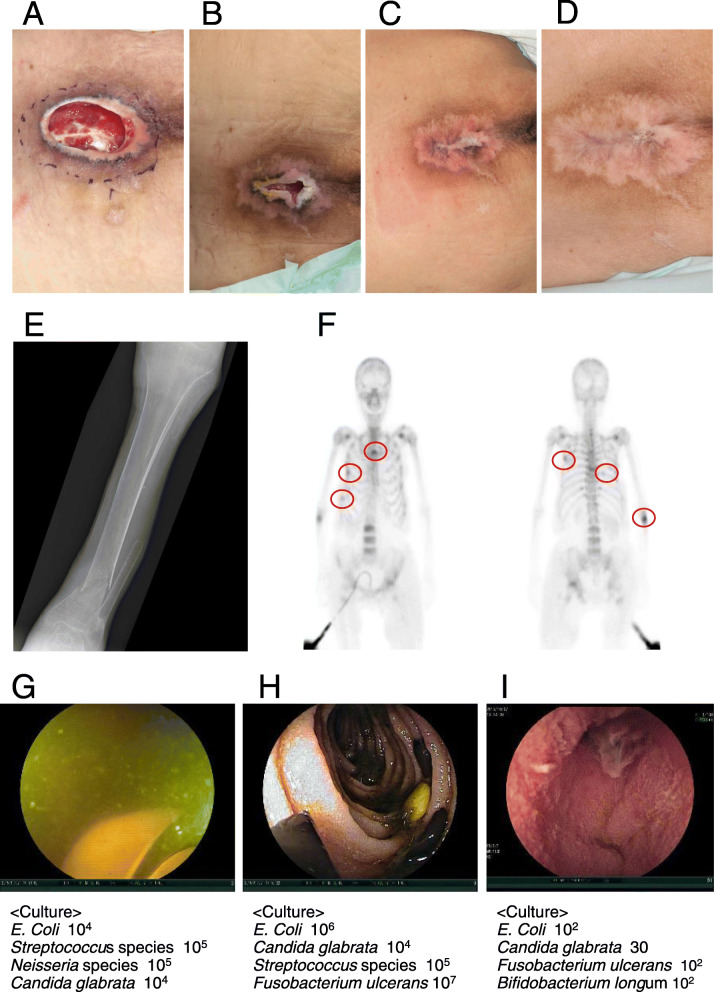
Patient images. a, b, c, and d Sacral pressure sore. a Day 0: sore 10.0 × 6.5 cm in size with an entrance of 6.0 × 3.5 cm; DESIGN-R score was D3 e3 s8 i0 g3 N3 P24 with a total score of 44. b Day 405 (i.e., 285 days after starting SDD for treating SIBO): reduced size of sore; DESIGN-R score was D3 e3 s3 i0 g1 n0 p0 with a total score of 10. c Day 742 (i.e., 617 days after staring SDD): healed sore; DESIGN-R score was d0 e0 s0 i0 g0 n0 p0 with a total score of 0. d Day 1057 (i.e., 932 days after staring SDD): no recurrence of the sore. e and f Osteoporosis and multiple fractures. e X-ray showing left tibial fracture. f Tc-99 m bone scan showing accumulation in multiple ribs, vertebrae, and right ulna. g, h, and i Endoscopic examination and results of bacterial culture of the upper digestive tract. All stomach, duodenum, and proximal jejunum samples were positive for *E. coli*. g Stomach. Food residue can be seen. Acid level was decreased to pH 7.0. h Duodenum. Food residue is evident. i Proximal jejunum. Flat villi and a jejunal ulcer are observed
